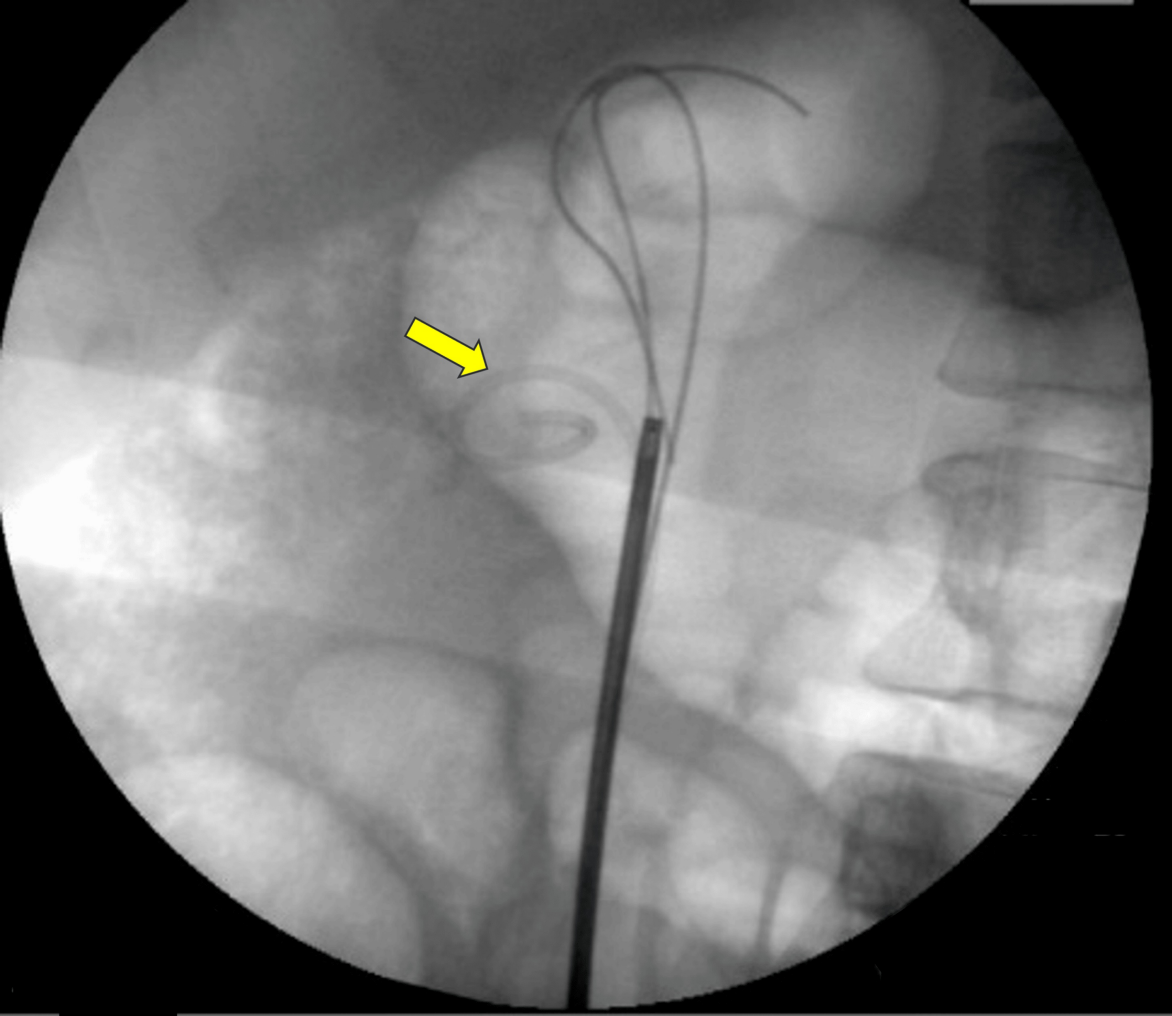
Intraoperative X-ray showing an attempt to gain access to the renal pelvis during failed stent removal (arrow).
